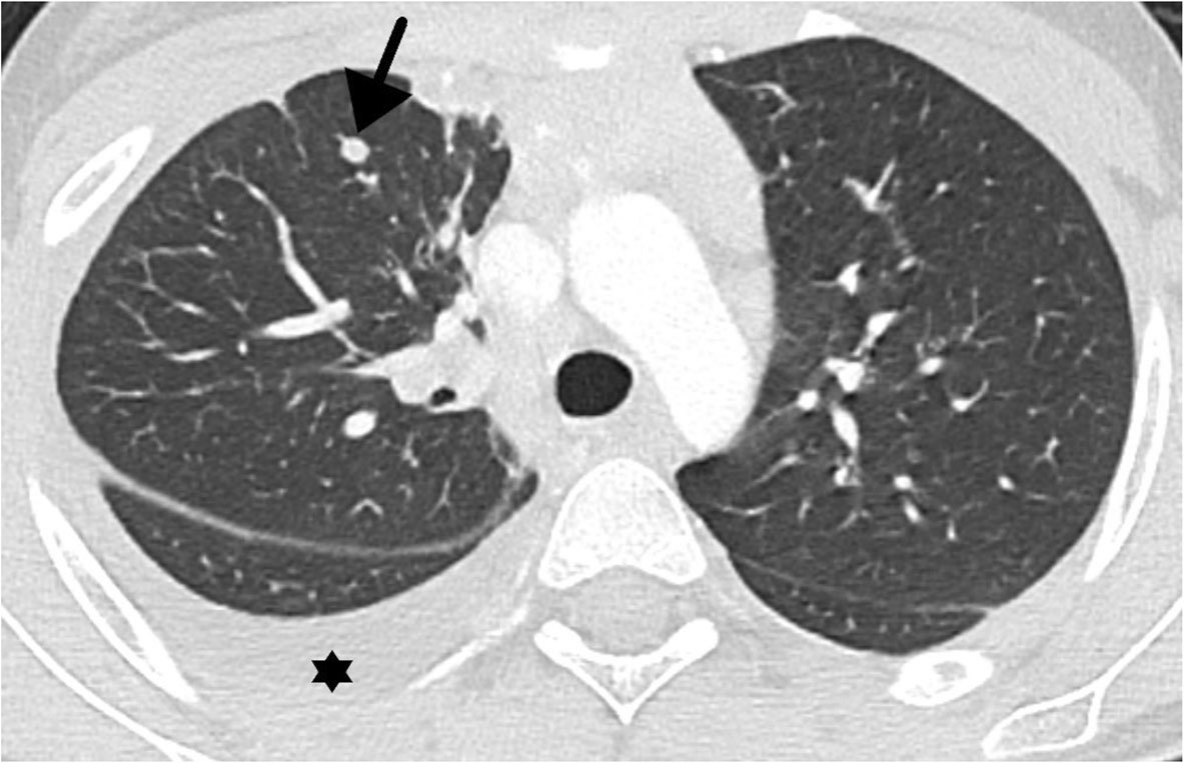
Atypical features in a 11-year-old girl with rt-PCR positivity for SARS-CoV-2 admitted due to polyarthritis and fever—unilateral lung effusion (*) and 2 solid nodules (arrow) as well as mediastinal and hilar adenopathies were documented. CT findings were thought to be associated with rheumatological disease
